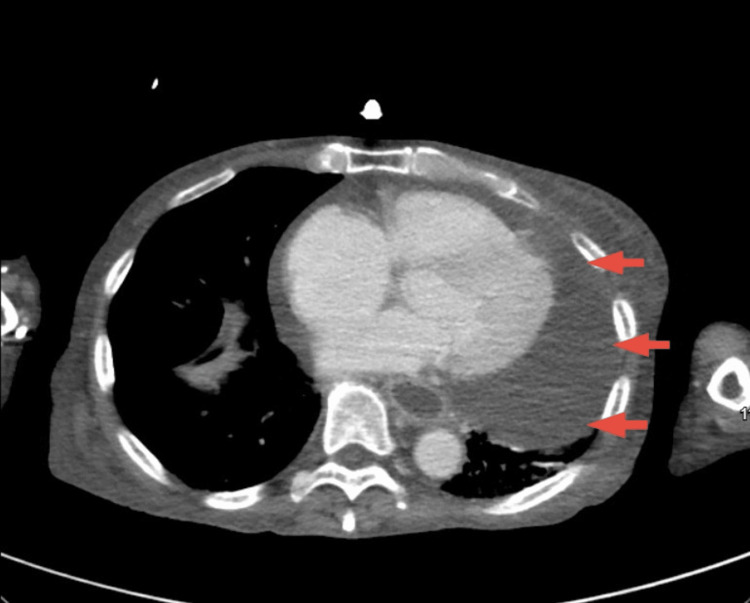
Computed tomography demonstrating a large circumferential pericardial effusion (arrows).
